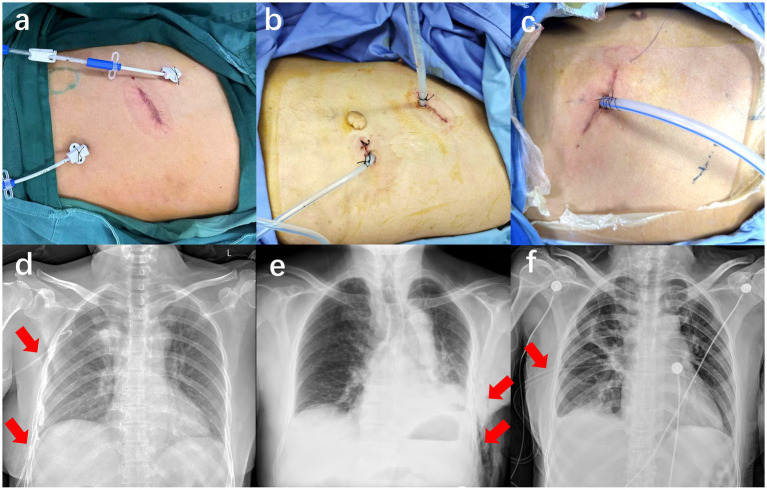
(a) Placement of two ultrafine 8F chest tubes, (b) Placement of one 22F chest tube and one 8F chest tube, and (c) Placement of one 24F chest tube after U-VATS for lung tumors. Postoperative thoracic cavity X-rays of patients treated with (d) two ultrafine 8F chest tubes, (e) one 22F chest tube plus one 8F chest tube, or (f) one 24F chest tube.
